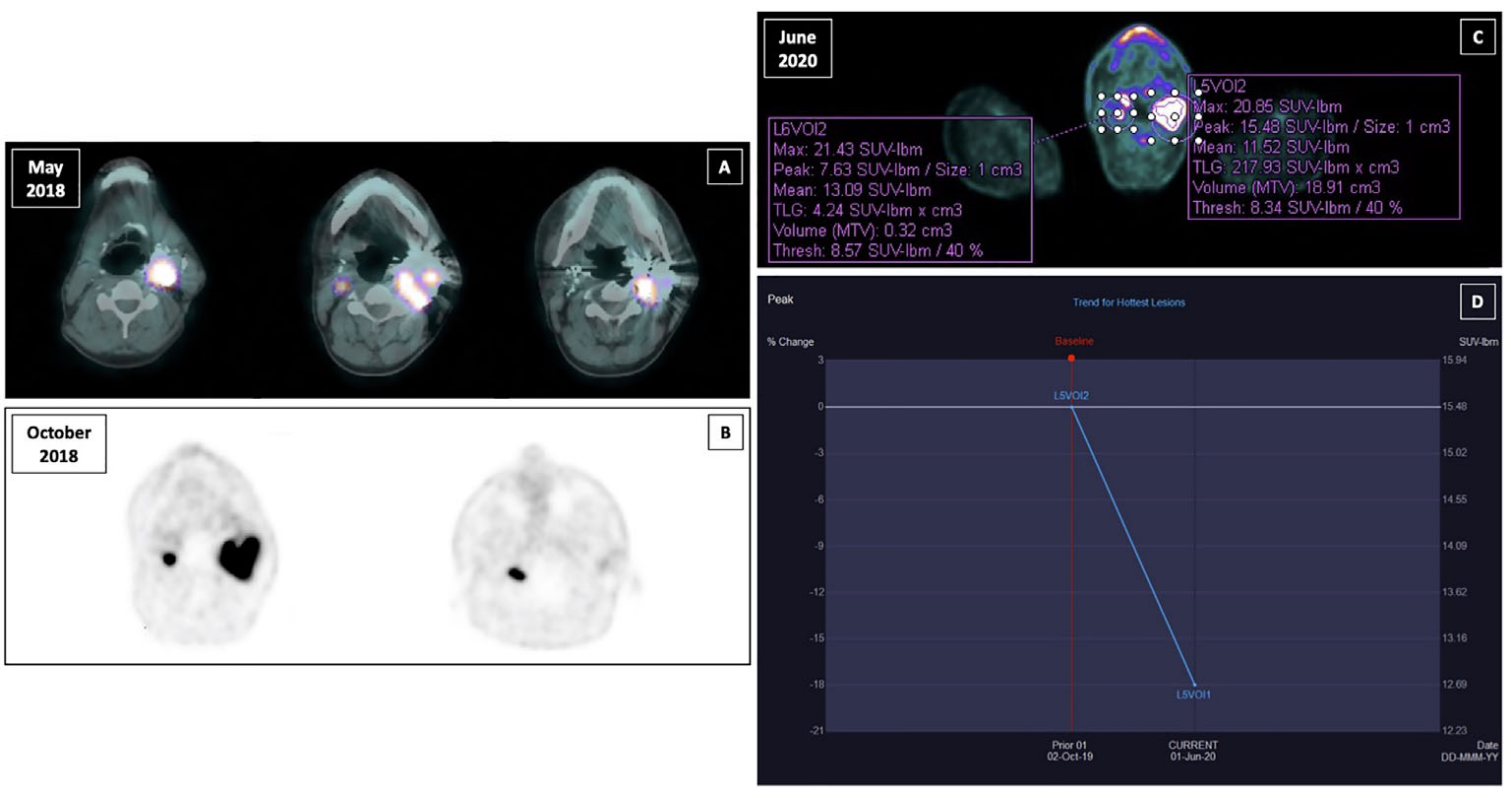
Functional imaging of right and left paraganglioma and response to sunitinib treatment assesed by PERCIST criteria. (A) 18F-fluoro-dihydroxyphenylalanine (18F-DOPA) positron emission tomography (PET)-computed tomography (CT) in May 2018; (B) 68Ga-DOTA-D-Phe1-Tyr3-Octreotate (68Ga-DOTATOC) PET-CT in October 2018; (C) 18F-fluorodeoxyglucose (18F-FDG) positron emission tomography (PET)-computed tomography (CT) with contrast medium performed after the third cycle of therapy in June 2020; (D) peak standardized uptake value corrected for lean body mass (SULpeak) trend for the hottest lesion between the two susequent 18F-FDG PET-CT (October 2020 and June 2020).
